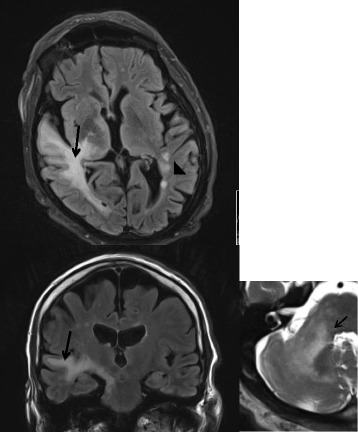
Brain MRI displaying PML features in a PCV patient treated with hydroxyurea. Axial and coronal T1-weighted sections displaying multifocal and confluent supra- and infratentorial lesions without contrast enhancement (*arrows*). There are also supratentorial punctate lesions (indicated by an arrow head). To the right T2-weigthed section displaying lesions in the right cerebellar hemisphere (*arrow*). No restricted diffusion
